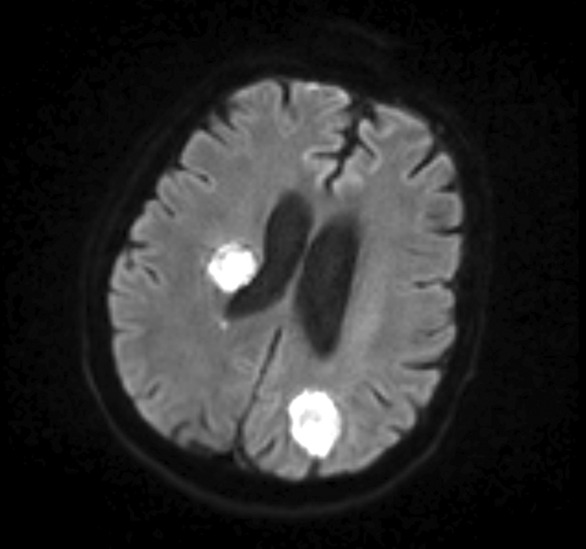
MRI of the brain revealing several intracranial ring‐enhancing lesions with surrounding edema suggestive of fungal abscess.
